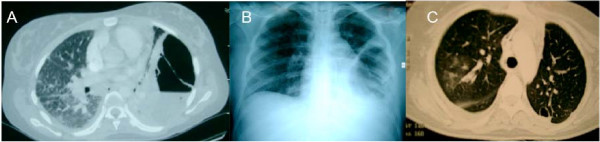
Initial thoracic CT-scan (A) and chest X-ray (B), thoracic CT-scan after chest tube and under mechanical ventilation (C).
